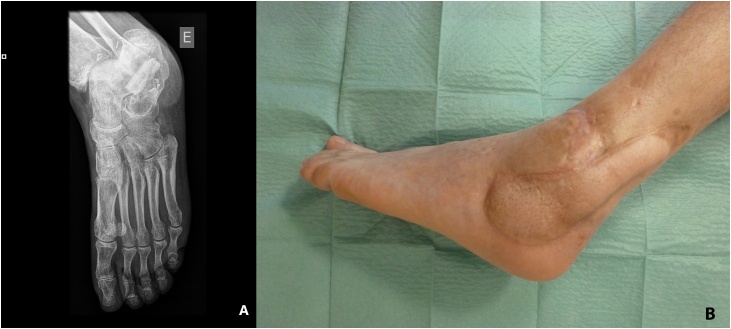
A – Radiographic appearance of the reconstructed defect 9 months postoperatively. B – Photography of the foot 12 months post-operatively.
